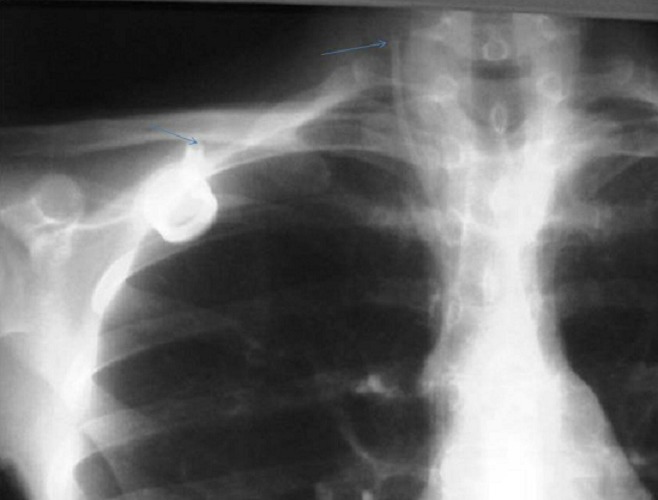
Radiographie thoracique de face on note la migration du cathéter au niveau de la veine jugulaire interne droite loin de la chambre (Flèches: bout du cathéter dans la VJI déconnecté de la chambre)
